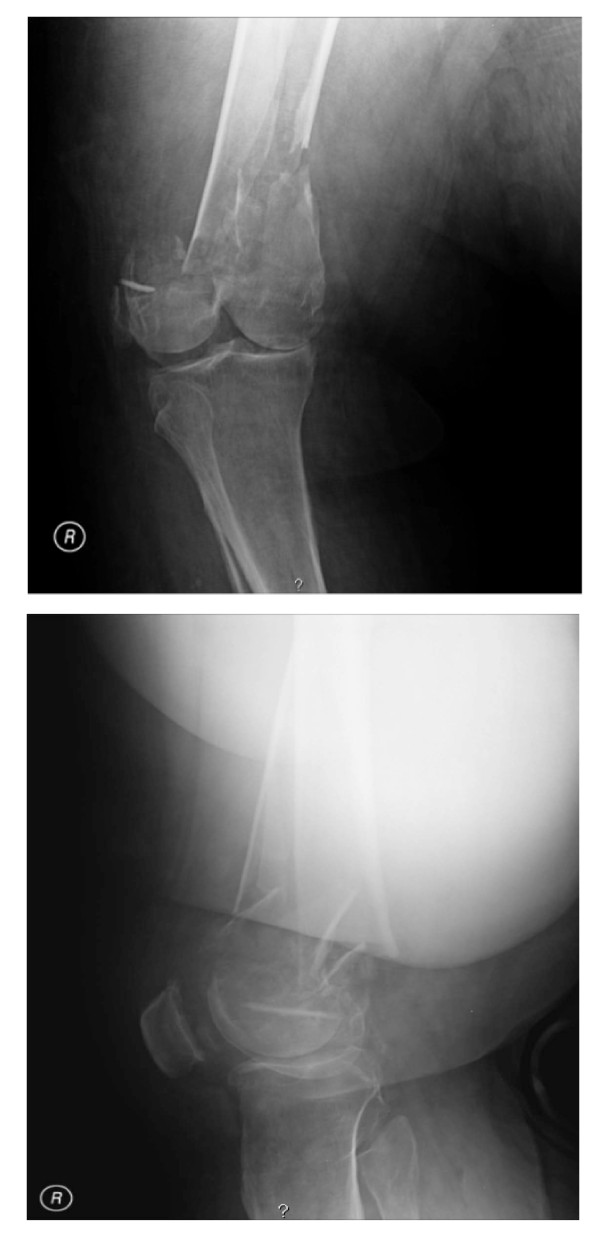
A-B Pretraction radiograph demonstrating distal femur fracture.
